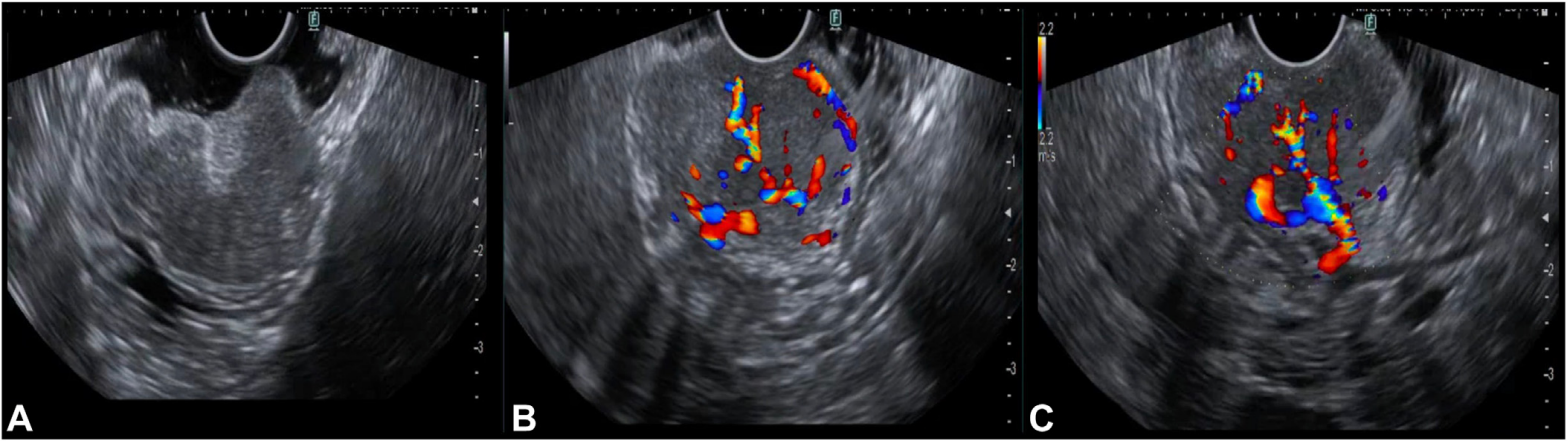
EUS view of the gastric subepithelial lesion. A, EUS revealed a large hypoechoic lesion with an irregular luminal surface seen within the submucosa, with focal infiltration into muscularis propria. B, The lesion shows diffuse vascular signal on the color Doppler. C, A large feeder vessel seen on EUS during color Doppler view.
